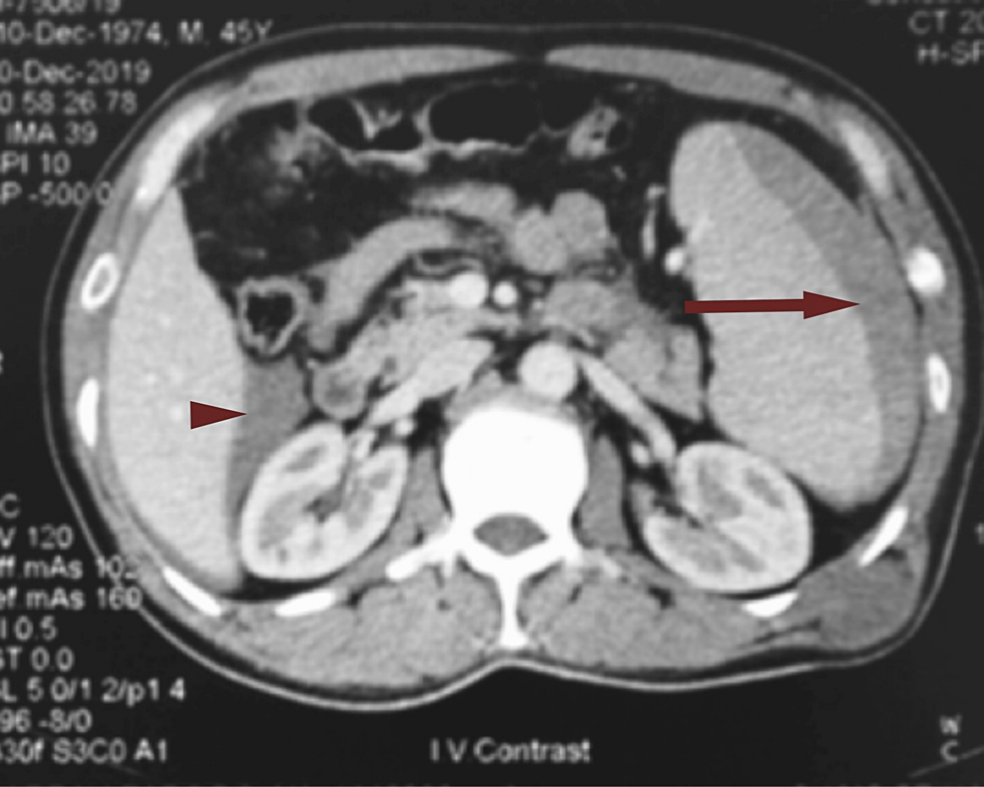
Contrast CT abdomen suggestive of a large subcapsular splenic hematoma (arrow) with moderate free fluid in perihepatic region (arrowhead).
